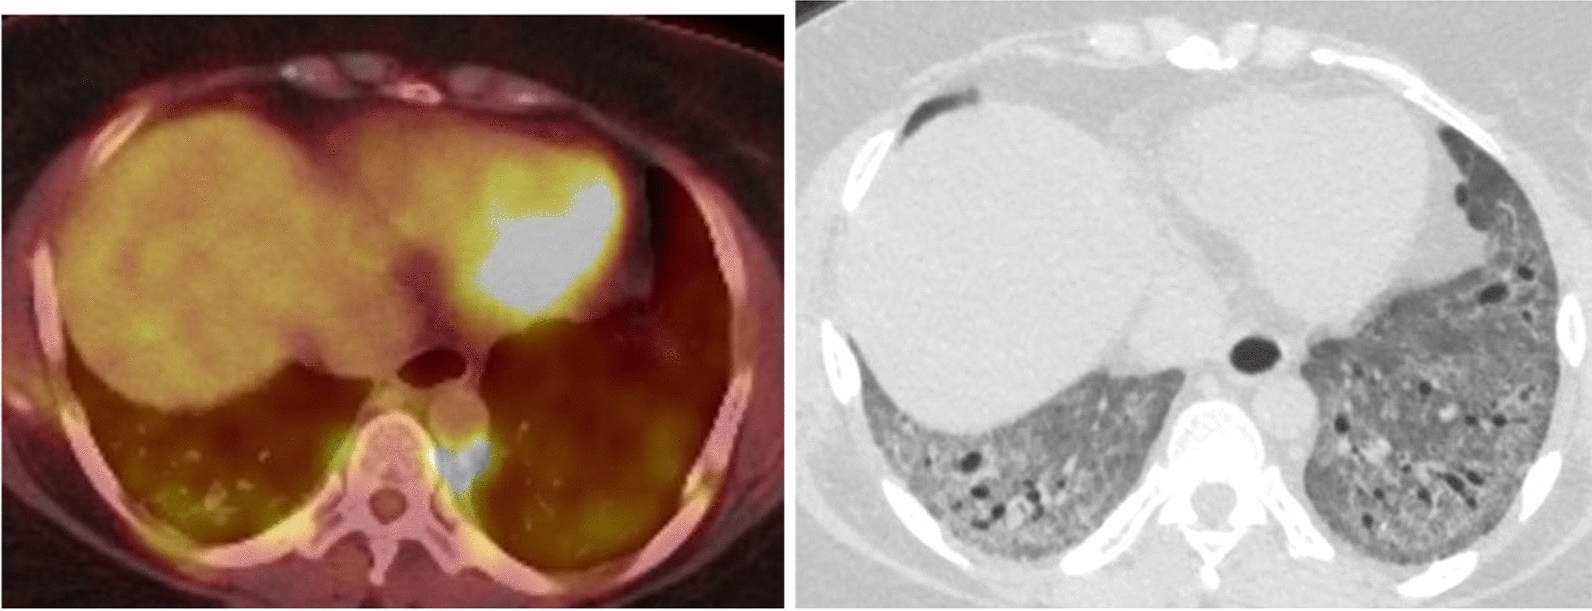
PET imaging with FDG demonstrates how both cancer cells and pulmonary fibrosis utilize glycolysis as an energy resource
